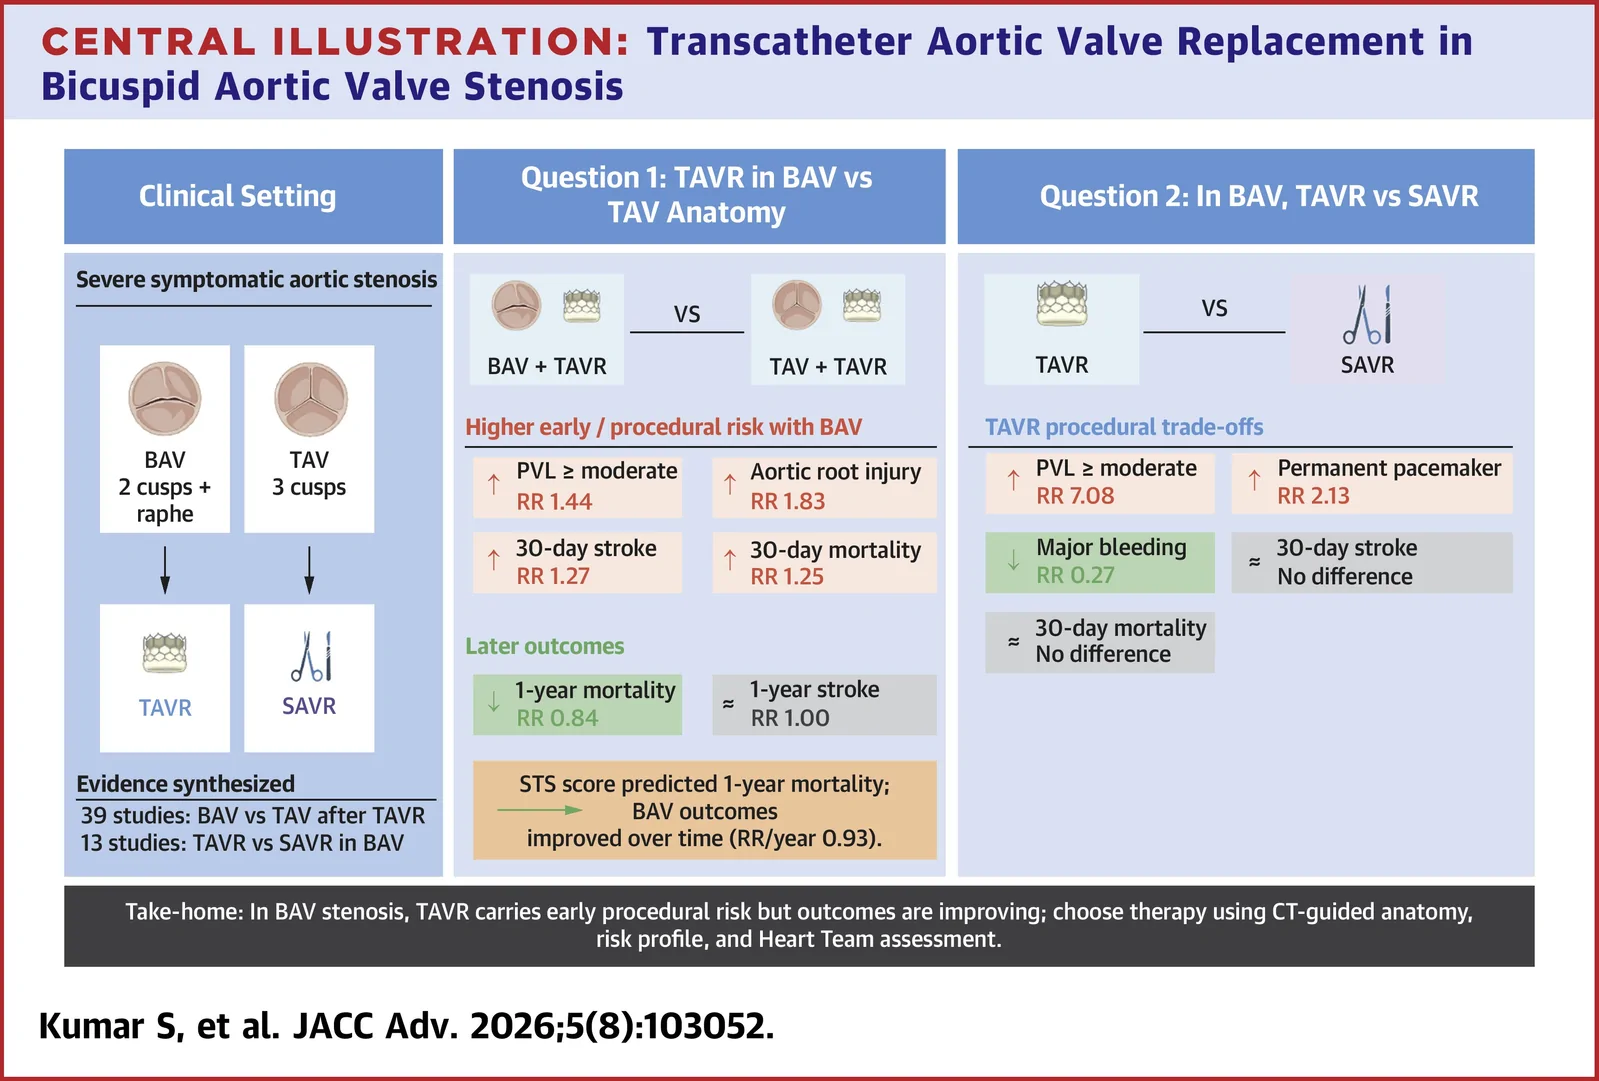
Transcatheter Aortic Valve Replacement in Bicuspid Aortic Valve Stenosis This systematic review and meta-analysis of 52 studies (295,615 patients) summarizes 2 clinical questions in severe bicuspid aortic valve stenosis. Compared with tricuspid anatomy, TAVR in bicuspid anatomy was associated with higher early procedural risk but lower 1-year mortality, with outcomes improving over time. Among patients with bicuspid stenosis, TAVR had higher paravalvular leak and pacemaker implantation but lower major bleeding than SAVR, with similar 30-day stroke and mortality, supporting individualized Heart Team decision-making. CT = computed tomography; SAVR = surgical aortic valve replacement; other abbreviations as in Figures 2, 10, and 12.
